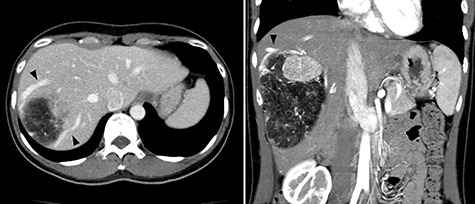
Abdominal computed tomography (CT) 5 years before referral to our hospital; enhanced CT revealed mixed density tumor measuring 12 × 10 cm in the right lobe of the liver; a peritumoral early drainage vein around the tumor (arrow heads) was visualized.
